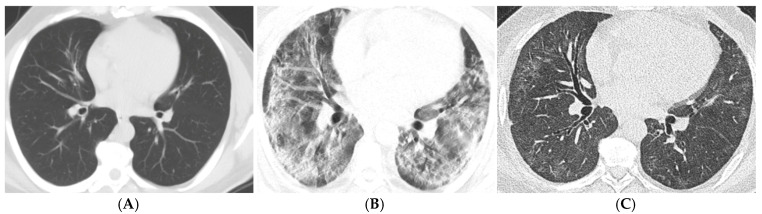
Axial CT scans of a 44-year-old male patient with moderate to severe COVID-19. (A) The images obtained two months prior to the onset of symptoms show that the lung parenchymal areas are completely normal, with no significant traction bronchiectasis observed. (B) The CT obtained 11 days after the onset of symptoms reveals ground-glass opacities, consolidation areas, and traction bronchiectasis bilaterally and in all lobes, with a CT severity score of 27. (C) The follow-up CT scan performed four months later shows patchy, blurry ground-glass opacities and markedly pronounced traction bronchiectasis that were not evident in prior scans before the COVID-19 infection.
